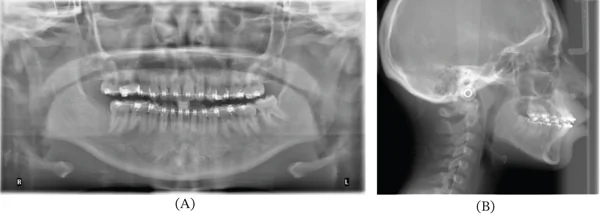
(A) Case #1 posttreatment panoramic radiograph. (B) Case #1 posttreatment lateral cephalogram.
